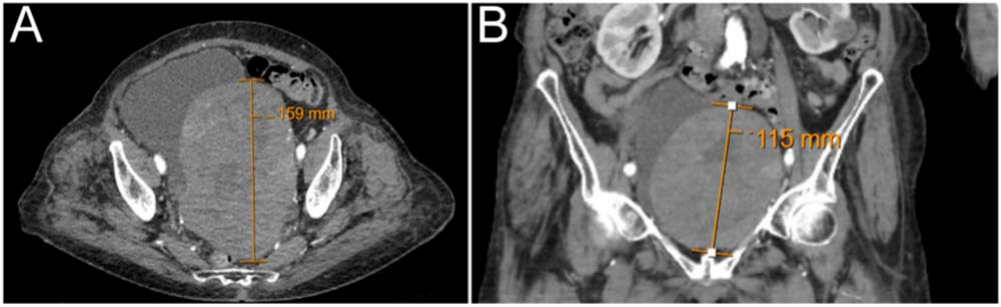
CT thorax, abdomen, and pelvis with contrast. Mass seen in the (A) axial view and (B) coronal view.
